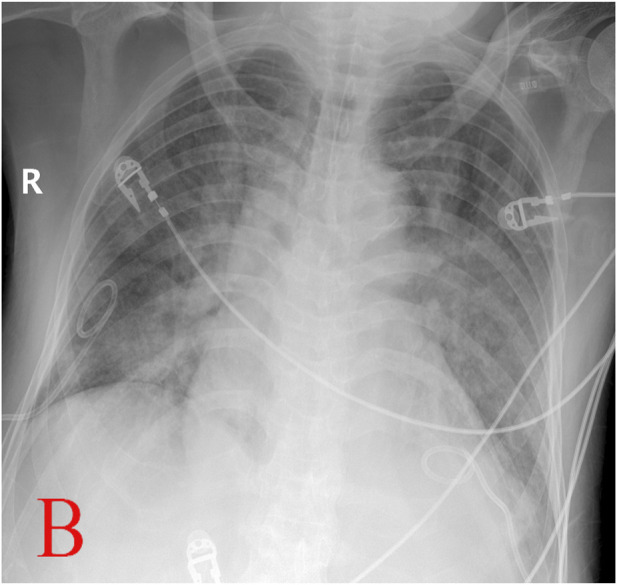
Chest X-ray after reintubation (B, Apr.15) (with permission from the patient.)
